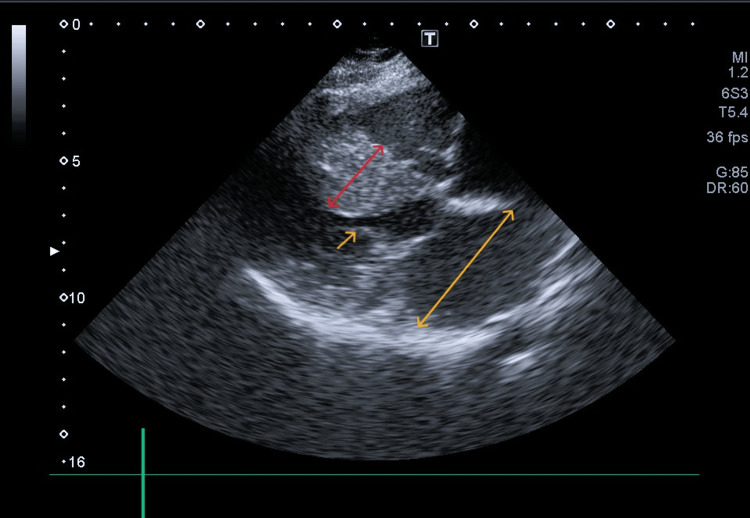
Extreme asymmetrical septal hypertrophy with systolic anterior motion of the anterior mitral valve leaflet Parasternal long-axis transthoracic echocardiographic view demonstrating marked asymmetric hypertrophy of the interventricular septum (red caliper), left atrial enlargement (yellow caliper), and systolic anterior motion of the anterior mitral valve leaflet (yellow arrow), contributing to left ventricular outflow tract obstruction.
